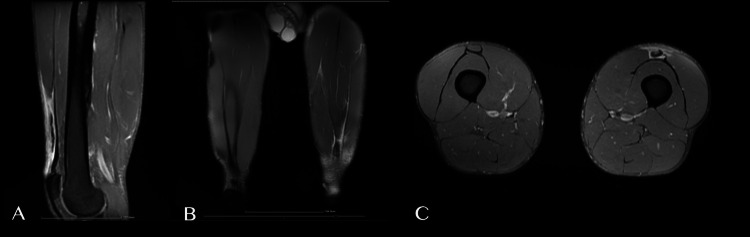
MRI T2-weighted sequence. (A) Sagittal plane. (B) Coronal plane. (C) Axial plane The images are compatible with a BAMIC grade 4c distal RF injury of the left thigh
